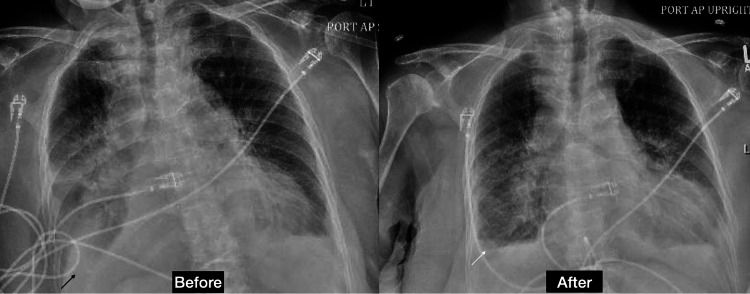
Chest X-ray before and after the second thoracentesis Moderate right pleural effusion (black arrow) and small left pleural effusion. Interval decrease of right side pleural effusion (white arrow).
